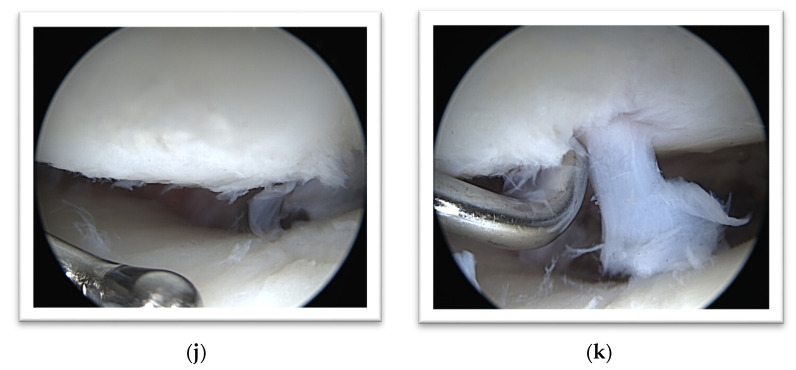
(a–i). Patella Dislocation. (a) Patella sunrise view, suggestive of MPFL and MPTL avulsion, a large osteochondral defect of the patella, and a displaced fragment in the lateral gutter. (b,c) MRI of the left knee, joint effusion, osteochondral fracture of the patella, displaced fragment in the lateral gutter, bone bruise of the lateral femoral condyle. (d–h) Intraoperative situation, osteochondral defect of the medial facet of the patella, before and after fragment fixation, small full-thickness osteochondral lesion of the lateral femoral condyle repaired with one chondral dart, partial-thickness chondral injury of the medial femoral condyle. (i) Postoperative patella sunrise view. (j,k). Arthroscopic re-evaluation three months postoperative: (j) the osteochondral lesion is healed and stable under probing, (k) the small remaining defect is filled with fibrocartilaginous tissue.
